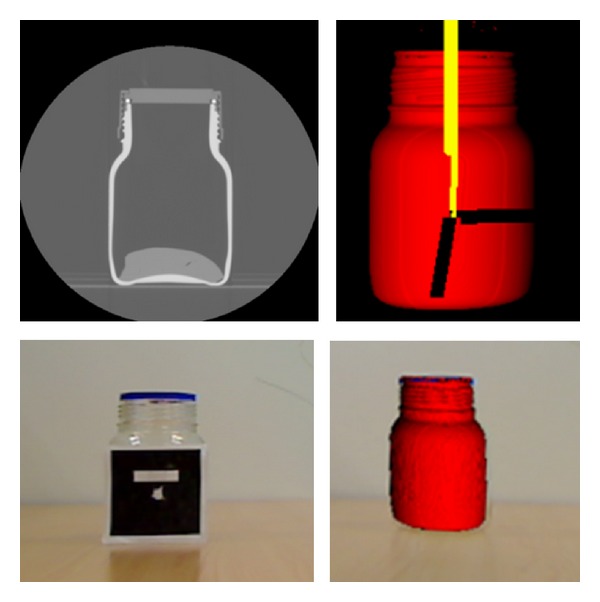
CT Jar images, 3D Jar model, marker, and registration and fusion of 3D virtual jar and real jar.
